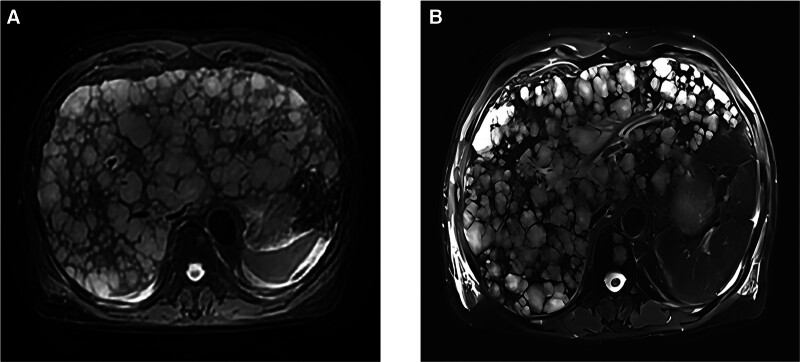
Abdominal MRI before and after treatment. (A) Patient’s abdominal MRI before chemotherapy. (B) Patient’s abdominal MRI after chemotherapy. MRI = magnetic resonance imaging.
